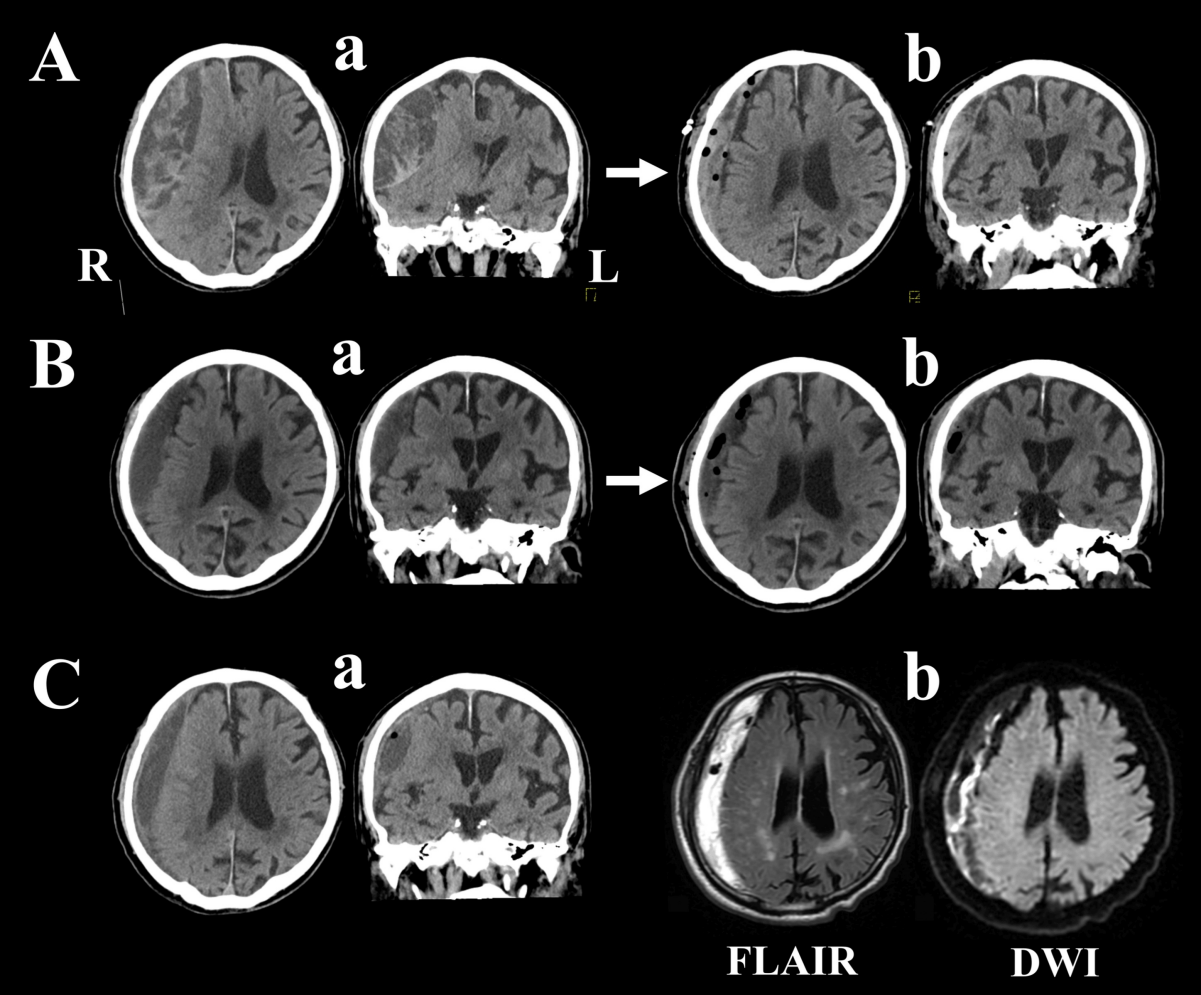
Before endoscopic surgery Aa: A computed tomography (CT) scan before the first surgery showed a thick subdural hematoma with heterogeneous density, causing strong compression to the cerebral cortex and midline shift; b: burr-hole irrigation and drainage contributed to a reduction of the hematoma volume, resulting in the release of brain compression and improvement of the midline shift. Ba: Head CT 20 days after the first surgery revealed re-accumulation of subdural hematoma and compression of the brain with midline shift; b: second irrigation and drainage were performed, contributing to a decrease in the hematoma volume and release of brain compression. Ca: Thirty days after the second surgery, a CT revealed further accumulation of the subdural hematoma, compressing the cerebral cortex; b: magnetic resonance imaging revealed heterogeneous signals along the edge of the cerebral cortex within the hematoma cavity on both fluid-attenuated inversion recovery and diffusion-weighted images, suggesting the presence of an organized inner membrane. FLAIR: fluid-attenuated inversion recovery; DWI: diffusion-weighted MRI
